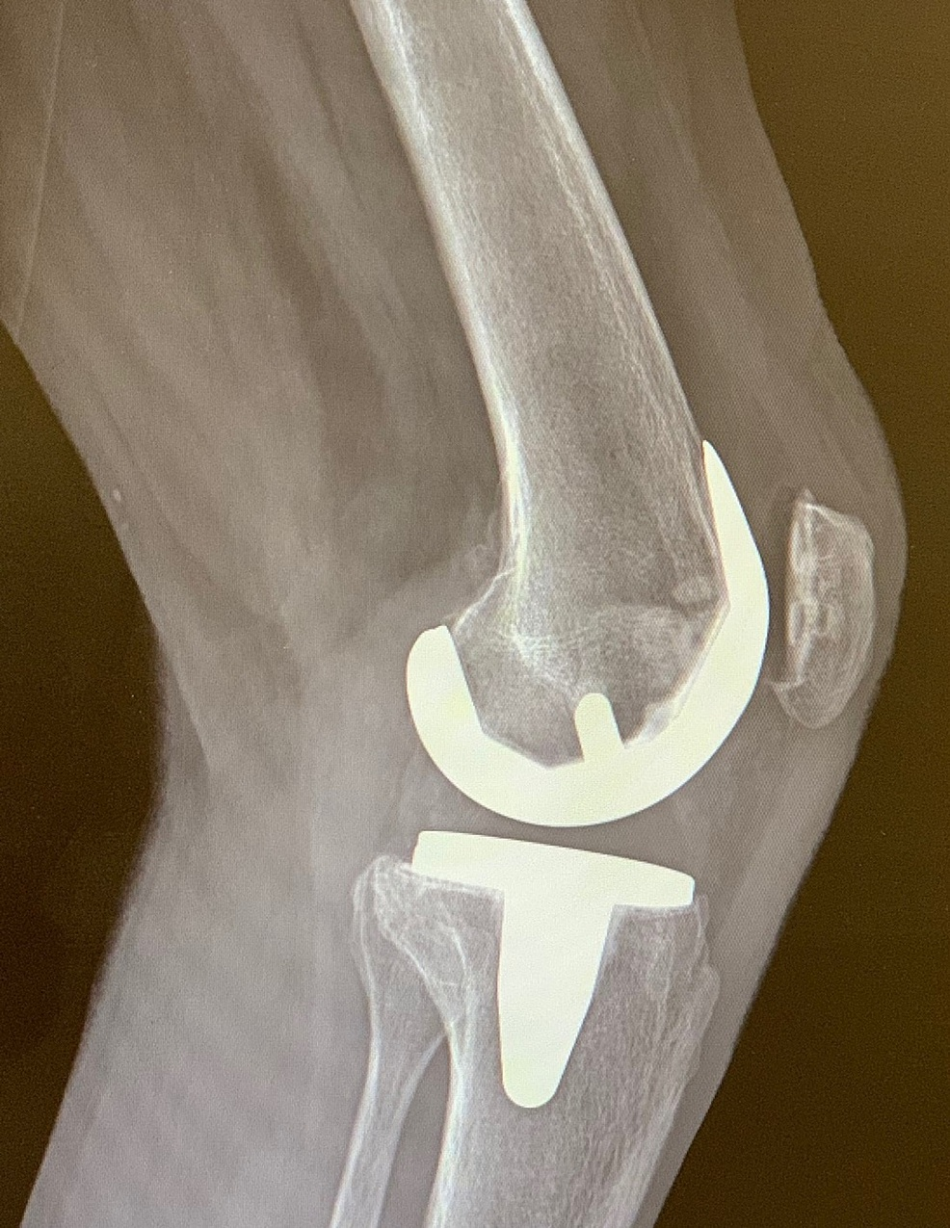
Lateral preoperative radiograph of the left knee demonstrating no obvious signs of aseptic loosening of the components.
